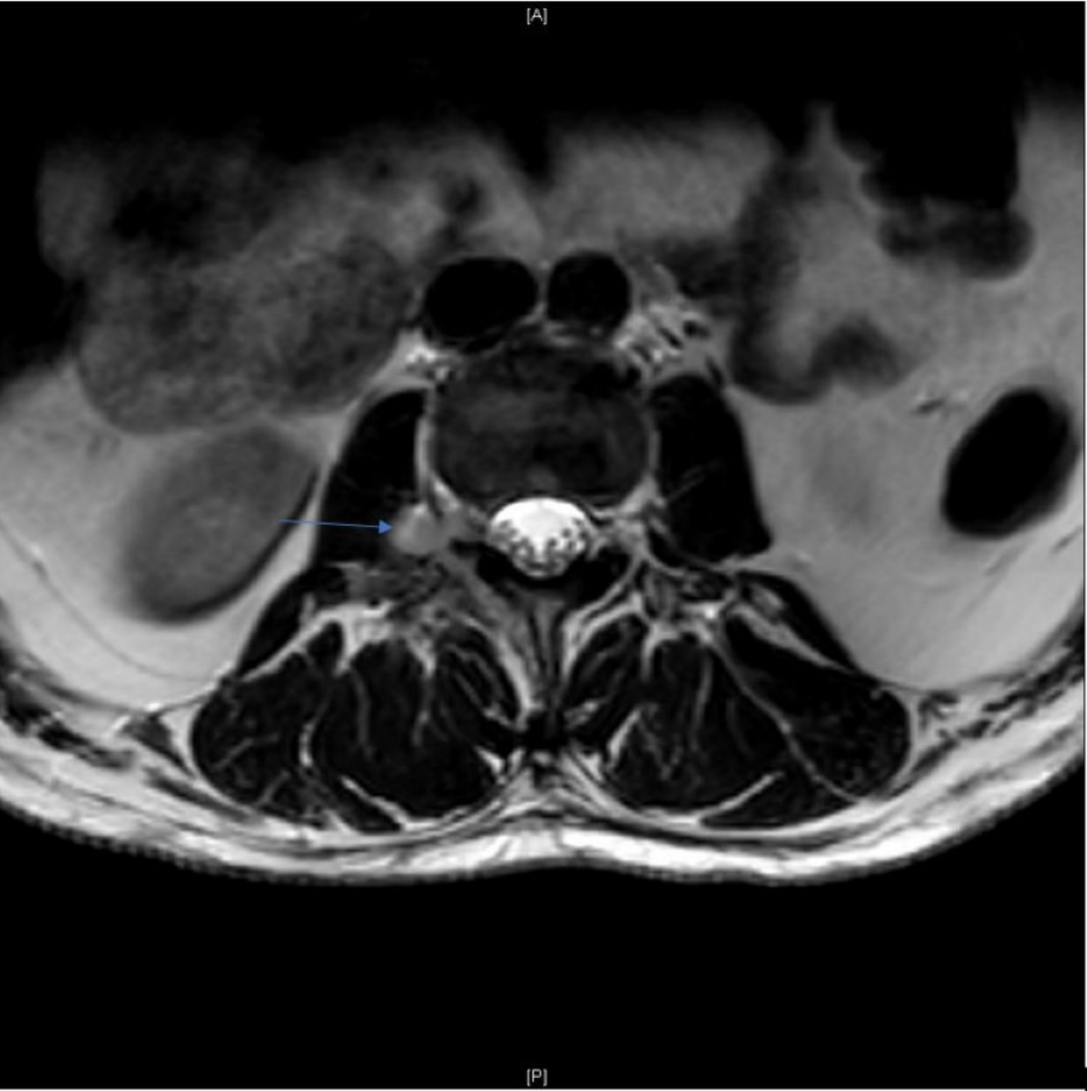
T2W Axial Magnetic Resonance Imaging (MRI) at L2/L3 Level on Day One of Admission. Gadolinium-enhanced T2-weighted axial MRI at the L2/L3 level showing epidural lesion in the right neural foramen (arrow) on day one of admission.
